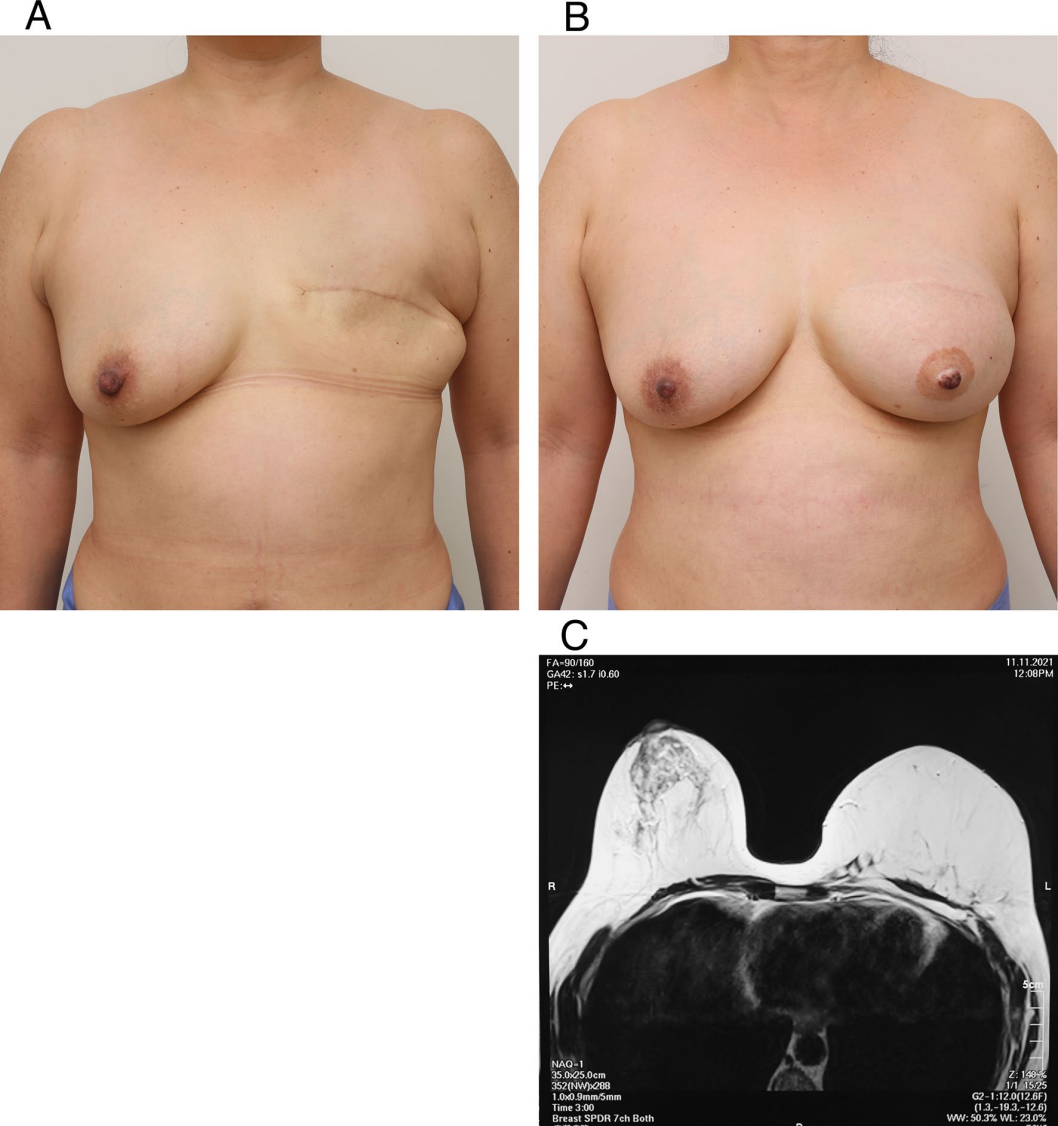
Clinical application of CAM-FG and MRI imaging after transplantation in unilateral case. (A) Pre-reconstruction view (after mastectomy) (B) Appearance of the breast at 4 years after two CAM-FG applications, 2 years after nipple and areola reconstruction. The nipple and areola were reconstructed using the Yanaga method^49,50^. (C) Transverse MRI image 3 years after transplantation. Parenchyma can be seen on the right side, but the left reconstructed side shows only adipose tissue. The adipose tissue shows up as white, and adipose tissue can be confirmed to have formed in all layers. In addition, blood vessels can be seen in the tissue. No fat necrosis, cysts, or calcifications were observed. Stable adipose tissue formation was observed in this or any of the other cases.
